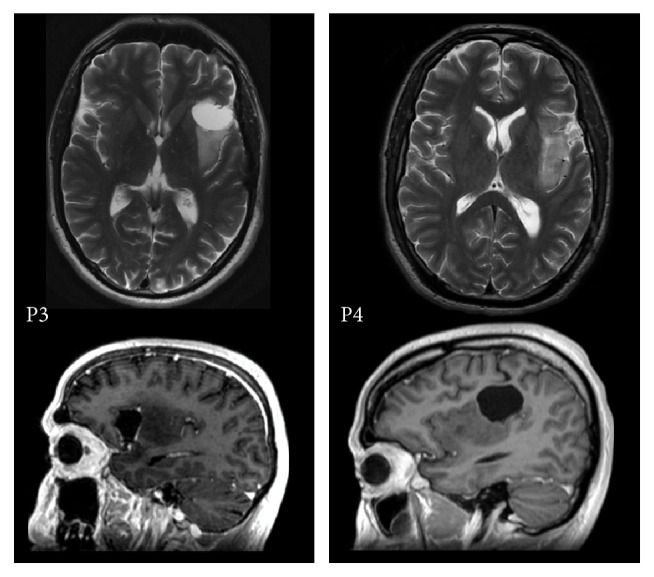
Postoperative MRI scans axial T2 weighted and sagittal T1 weighted P3 and P4.
